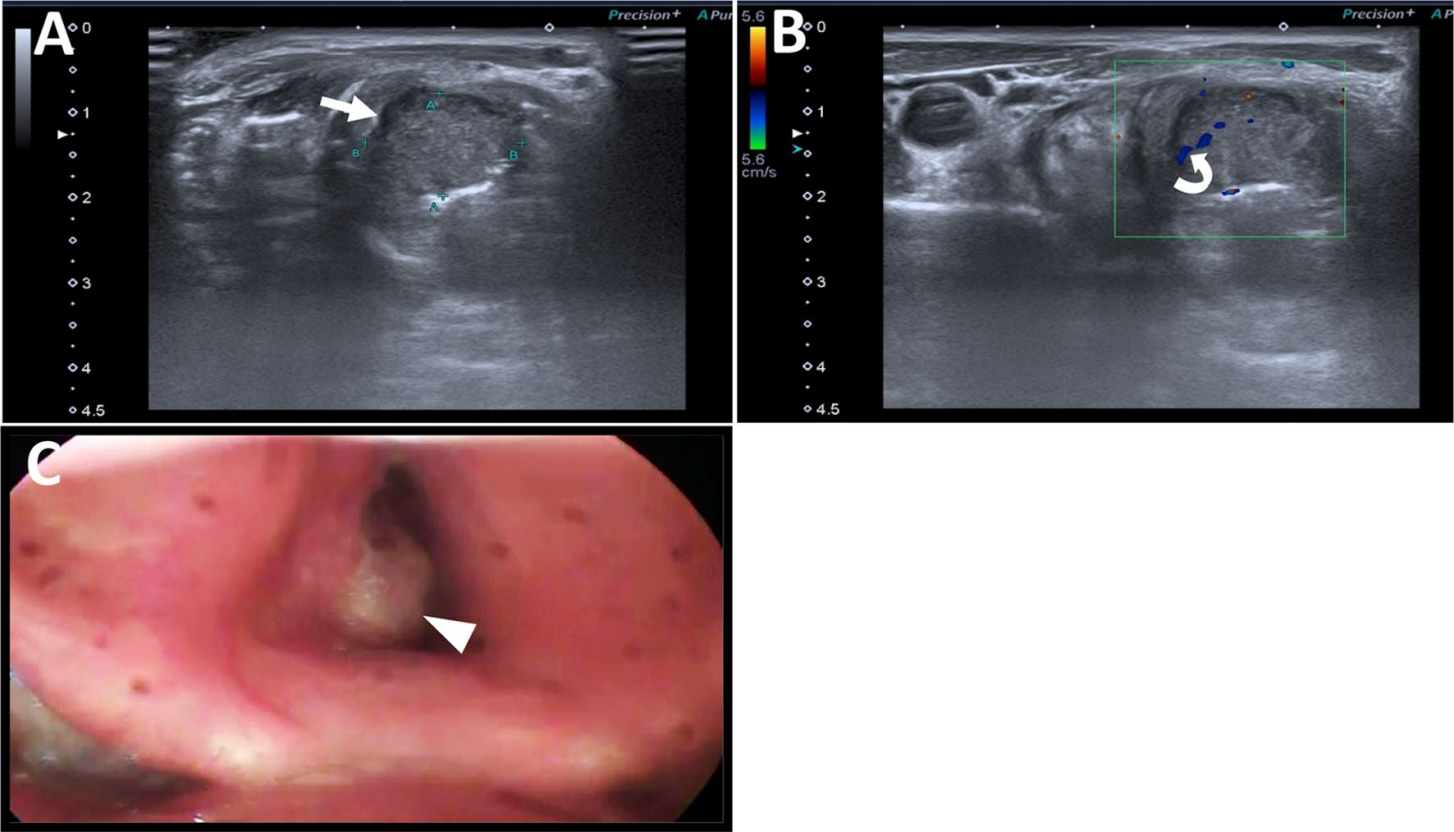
A 73-year-old male heavy smoker presented with hoarseness of voice, dysphagia, and stridor. A Laryngeal ultrasound right lateral axial view with right thyroid cartilage (asterisk) as anatomical landmark, shows a large soft tissue isoechoic mass lesion (arrow) almost completely replacing the right VC with ill-defined lateral portion of the right thyroid cartilage, indicating invasion. No related cervical LNs were detected. B Colored Doppler sonographic images revealed that the lesion has an internal color signal (curved arrow) denoting its hypervascularity. C Flexible laryngoscopy of the same patient shows the lesion in the posterior part of the right vocal cord (arrow head). The anterior is at the bottom of this figure. The flexible laryngoscopy confirmed the sonographic findings, yet it is limited in estimation of the proper size and extension of the subglottic component
